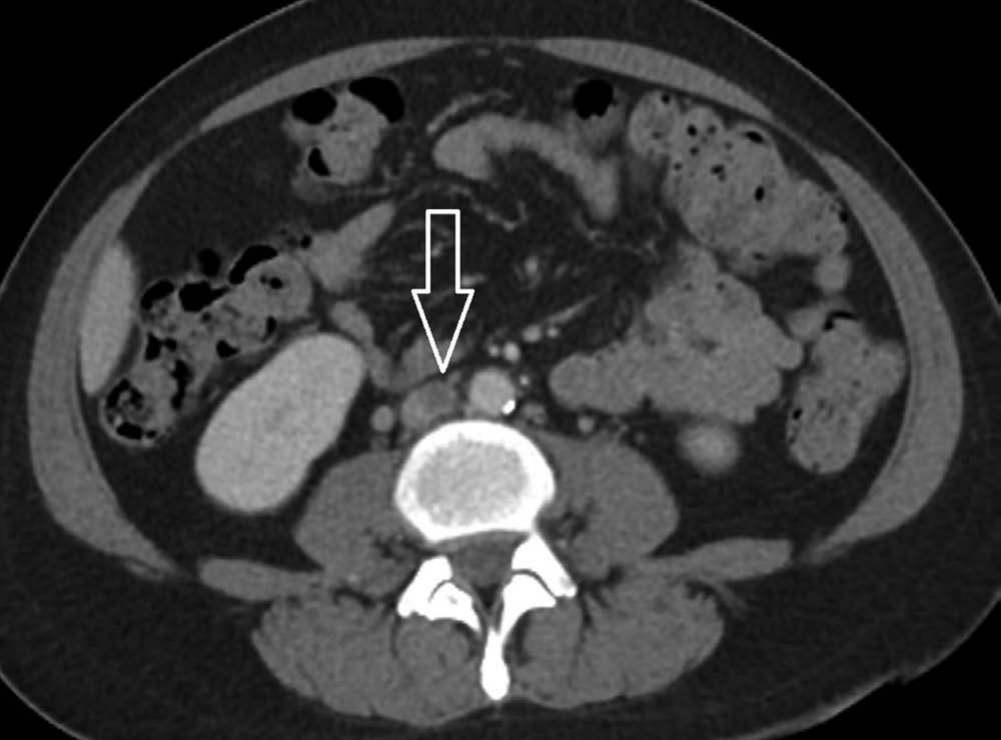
Axial image of computed tomography showing thrombosis (arrow) in the inferior vena cava.
